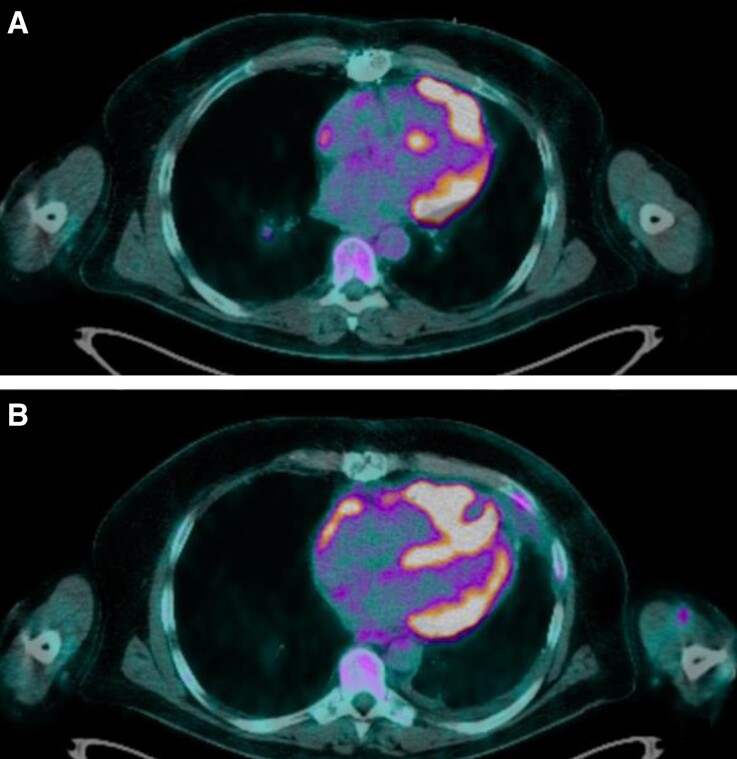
Evolution of lymphoma in 18 fluorodesoxyglucose (FDG) positron emission tomography scan. (*A*) Positron emission tomography 4 cavity plan initial. (*B*) FDG PET-scan 4 cavity plan re-evaluation after treatment with rituximab.
